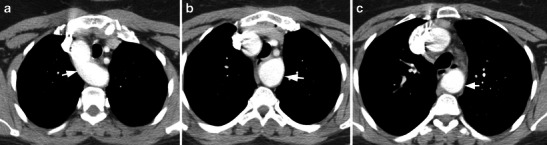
Circumflex right aortic arch. a Axial CT image shows a right aortic arch (*arrow*), which extends behind the oesophagus to reach the side. b Axial CT image at a lower level shows the arch has now reached the right (*arrow*) and continues as the descending aorta on the left. c Axial CT image at a lower level shows the left descending thoracic aorta (*arrow*)
